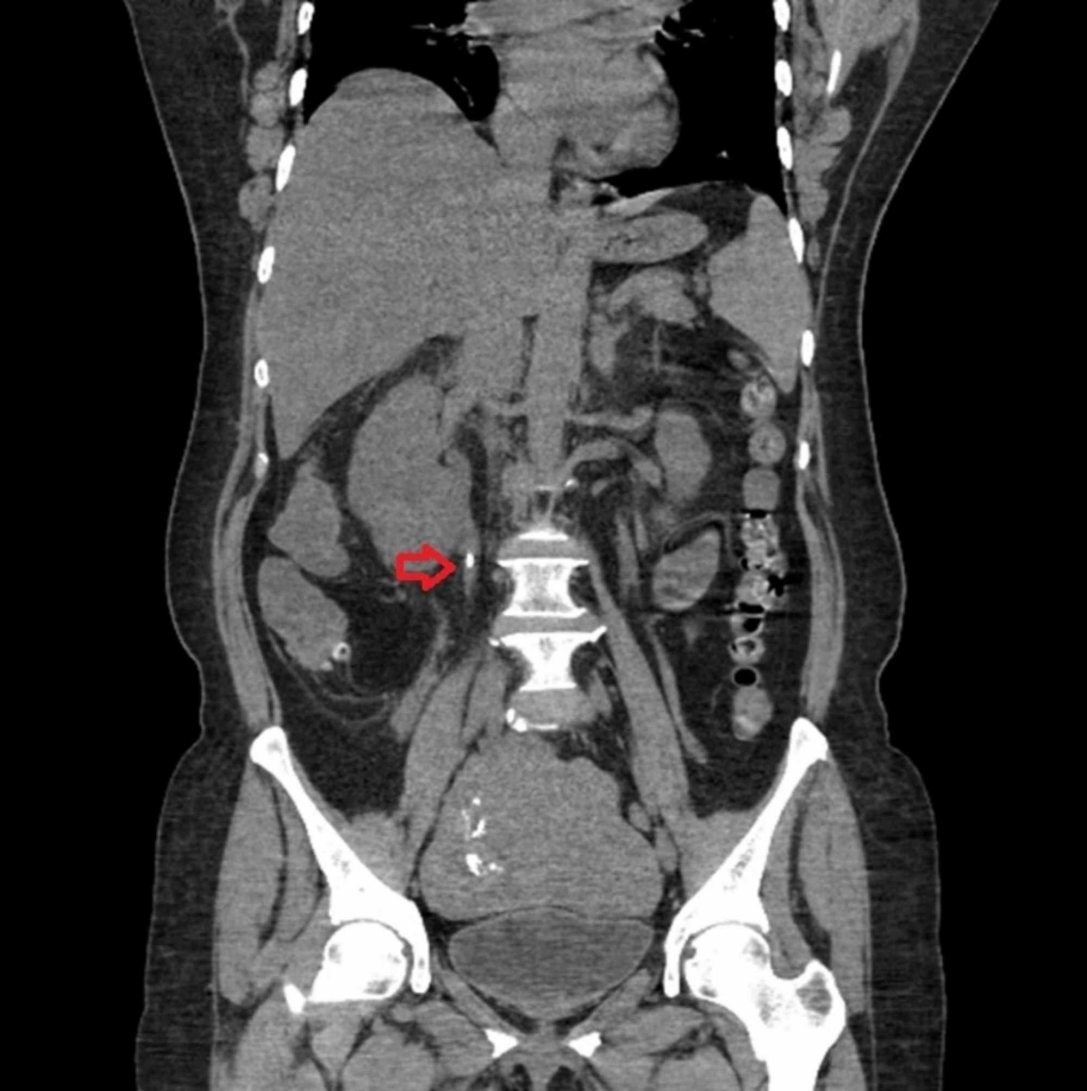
Computed tomography of the abdomen with contrast showing right-sided proximal ureteric renal calculi (red arrow)
